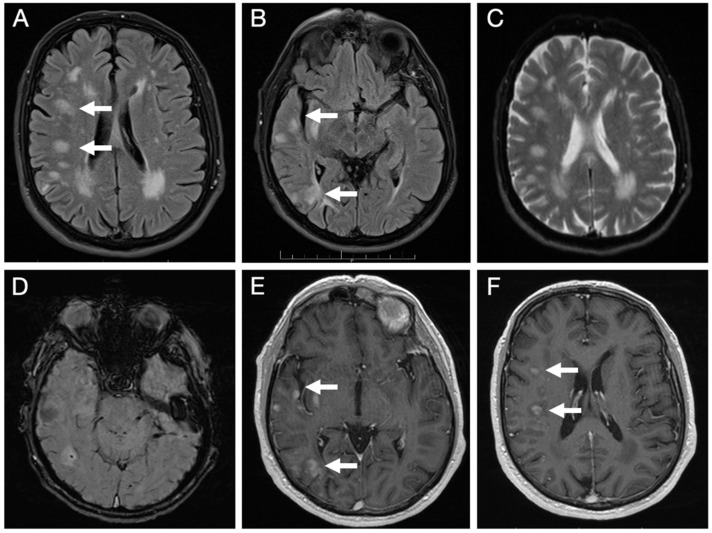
Initial brain MRI on axial FLAIR sequences shows multiple foci of hyperintense signal abnormalities in the subcortical and juxtacortical white matter of the right hemisphere (A,B); with facilitated diffusion (C); areas of signal loss compatible with microhemorrhages on SWI sequence (D); and patchy, nodular enhancement on post-contrast T1-weighted images (E,F).
